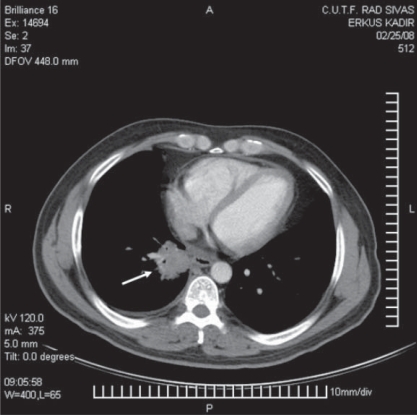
Panoramic radiograph of right lung of current case. Arrow indicates the enlargement tumor appearence
